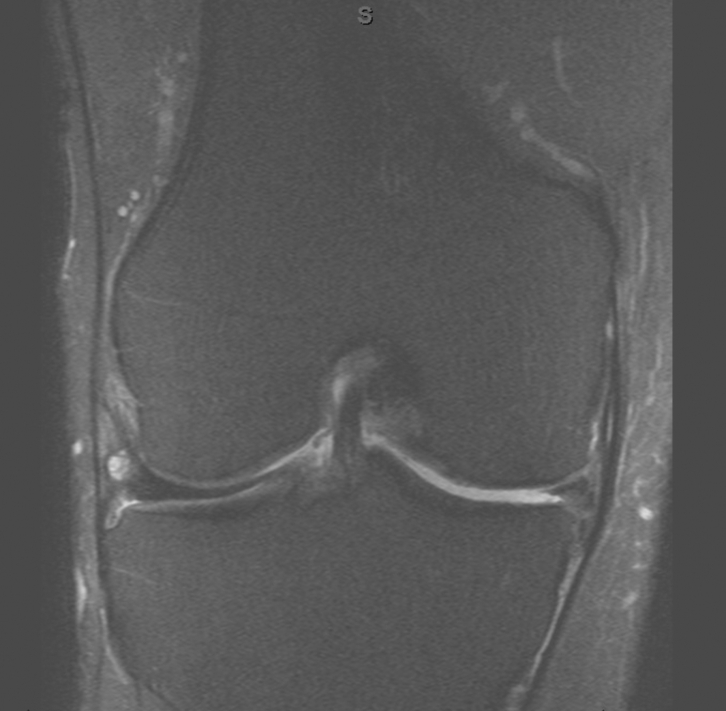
Magnetic resonance imaging of the left knee showing medial compartment chondromalacia.
